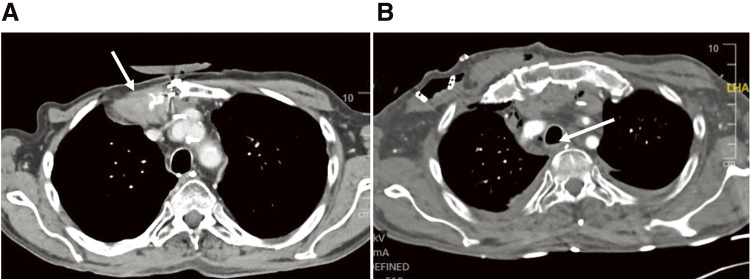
Postoperative CT. (A) The pectoralis major muscle was inserted in the thoracic cavity through the third ICS with a partial chest wall resection (arrow). (B) The posterior wall was competed and the fistula was completely removed (arrow). ICS, intercostal space
